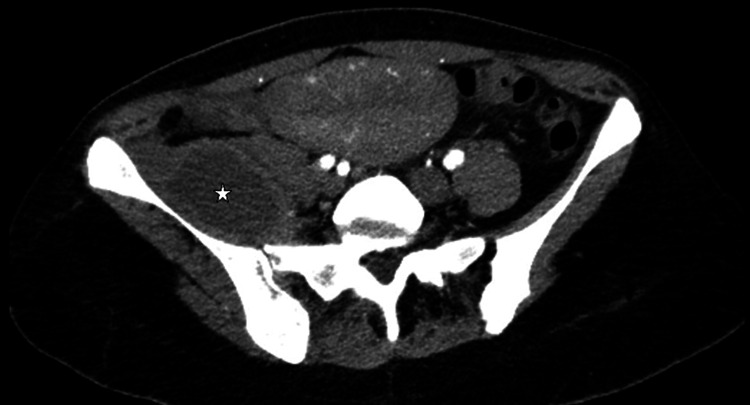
CT scan showing a psoas-infected hematoma in contiguity to the right sacroiliac joint The hematoma is marked with a star.
